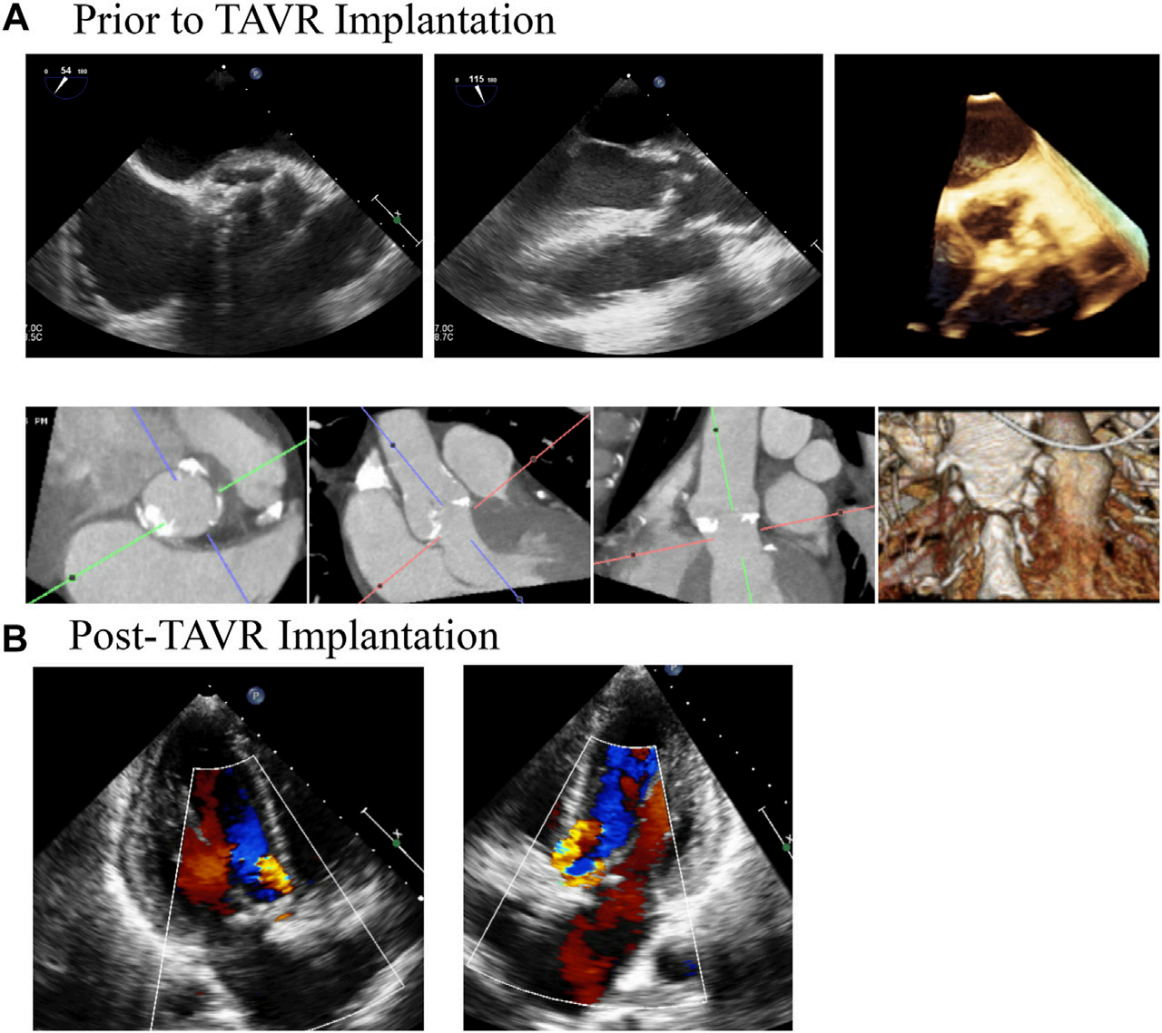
Medical Imaging of Patients Aortic Valve Demonstrates Clinically Significant Paravalvular Post TAVR Implantation. A patient was referred for TAVR for severe aortic stenosis. (A) demonstrates TEE and CT imaging of the aortic prior to the procedure. The patient had trace aortic insufficiency prior to the procedure. Following the procedure (B), the patient was found to have moderate paravalvular regurgitation that persisted for at least 1 year following the procedure, as demonstrated by the follow up TTE.
